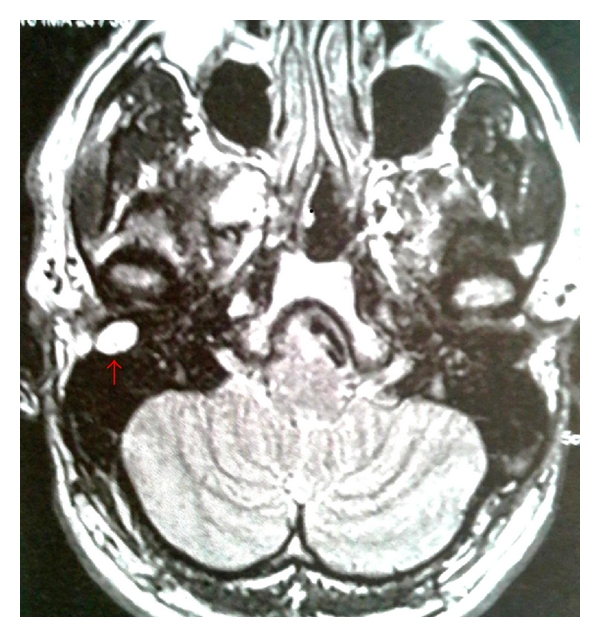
Axial T2-weighted MRI image. A well-bordered lesion was detected in the right external auditory canal. After intravenous gadolinium injection, the lesion had high signal intensity on T2-weighted image.
